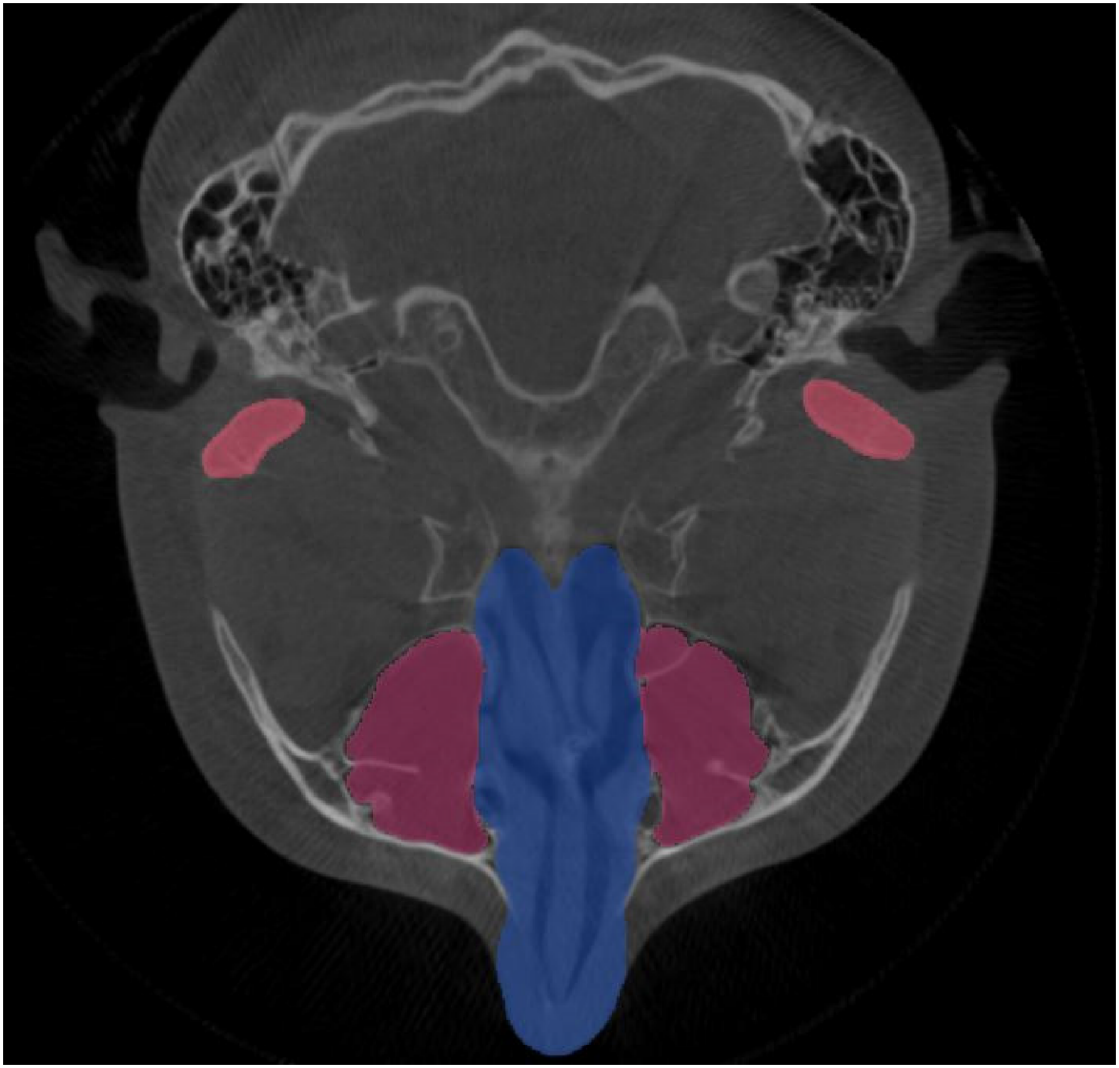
AI detection of various anatomical landmarks in the axial view of CBCT; the maxillary sinus, nasal cavity, and condyles.
